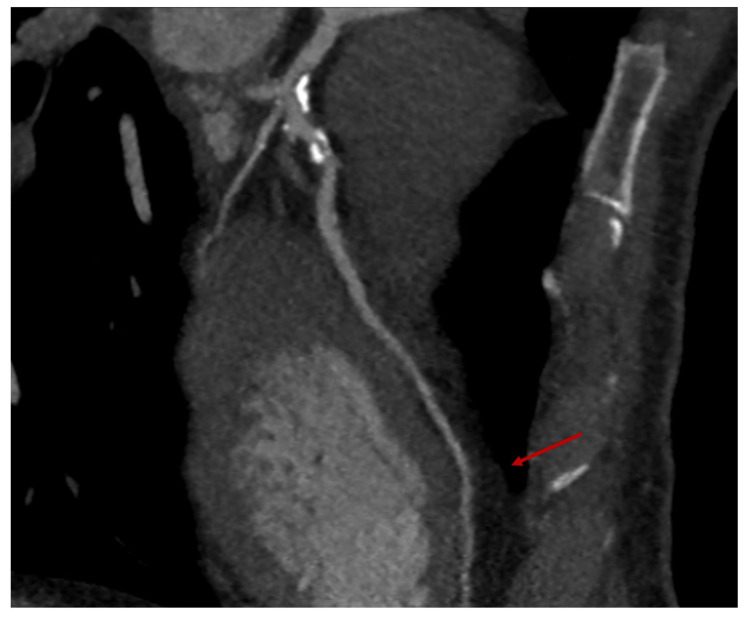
The coronary multislice computed tomography (MSCT), depicting—red arrow—the intramyocardial course of the left anterior descending (LAD), which represents a fundamental exclusion criteria prior to minimally invasive coronary surgery (MIDCAB).
